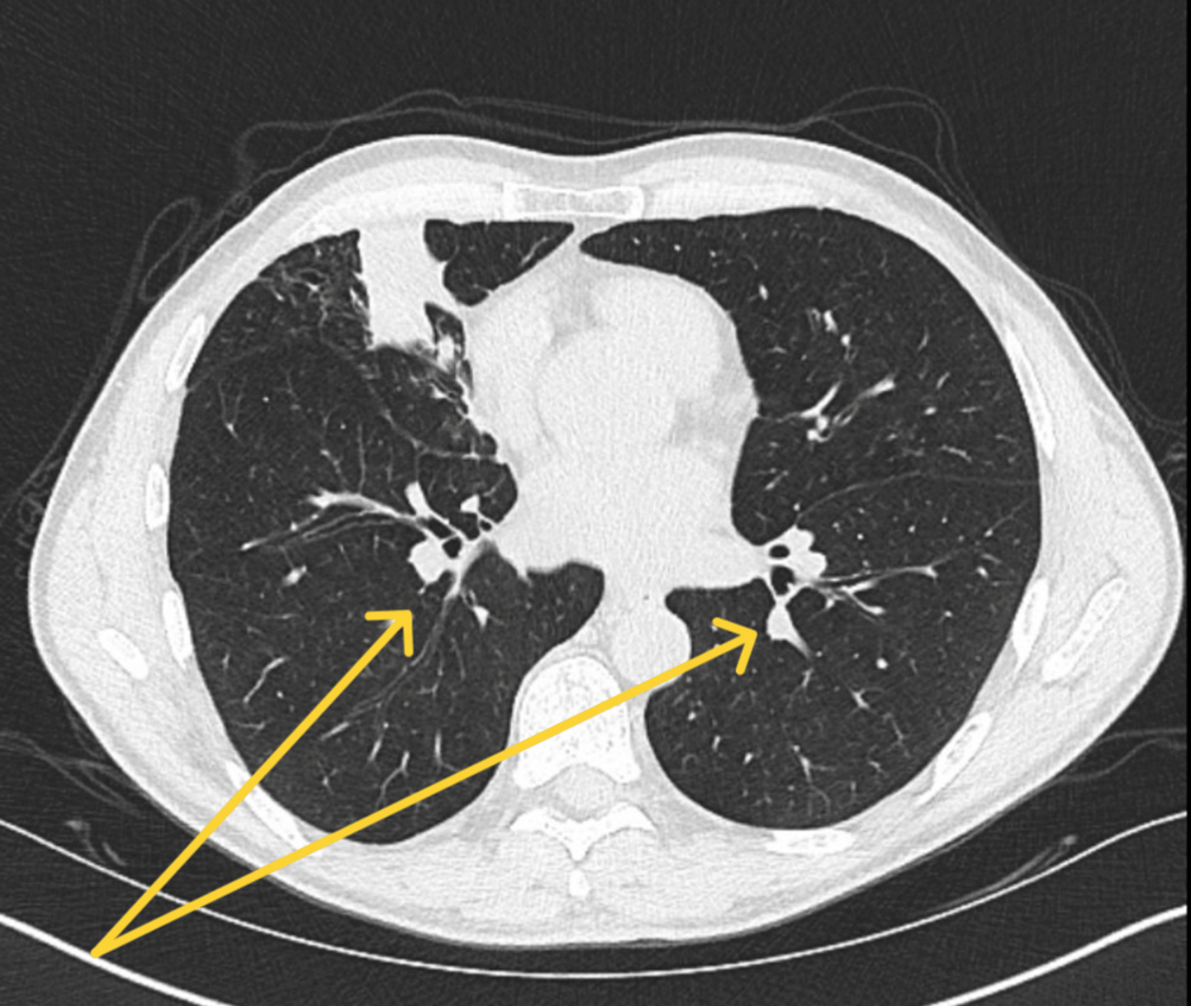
CT Chest With Contrast Yellow arrows: low-density airspace opacities present within the left upper lobe and right middle lobe, with a branching-like appearance.
